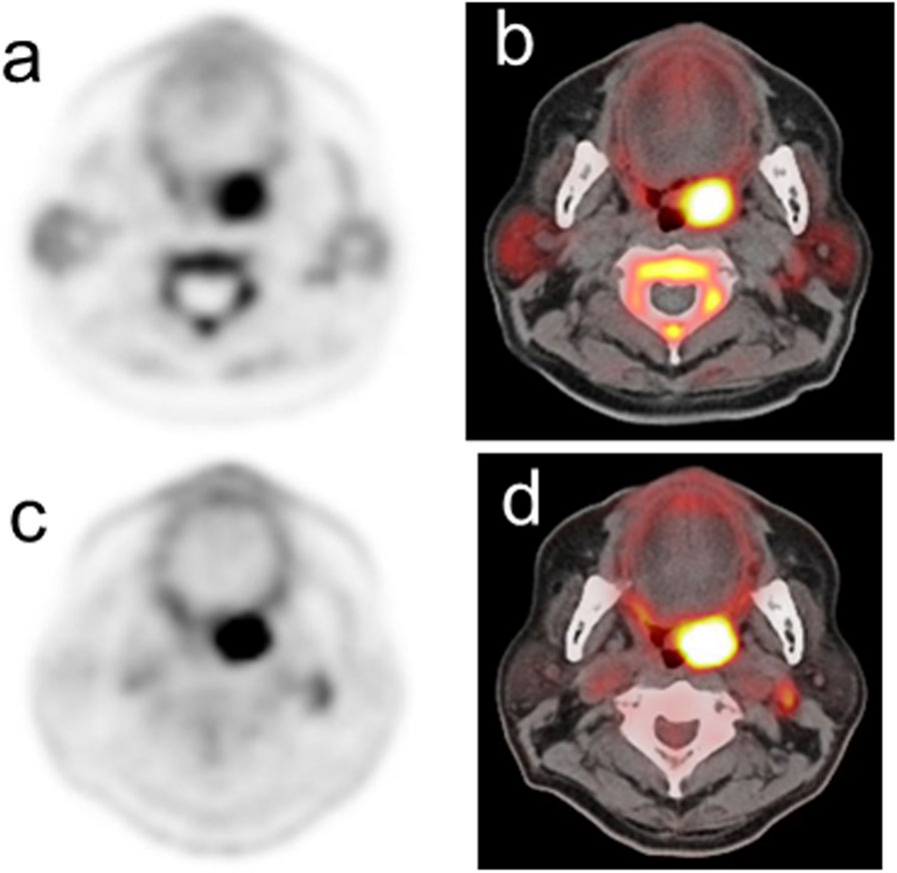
PET/CT images from a 67-year-old male diagnosed with p16-positive oropharyngeal squamous cell carcinoma in the left palatine tonsil. Transverse ^11^C-4DST PET image (a) and fused PET/CT image (b) show an increased uptake in the primary tumor (SUVmax = 7.41, homogeneity = 0.344, entropy = 2.130, SRE = 0.948, LRE = 1.220, LGZE = 0.00676, and HGZE = 199.90). Transverse ^18^F-FDG PET image (c) and fused PET/CT image (d) also show an increased uptake in the primary tumor (SUVmax = 14.42, homogeneity = 0.206, entropy = 2.230, SRE = 0.979, LRE = 1.080, LGZE = 0.00180, and HGZE = 867.60)
